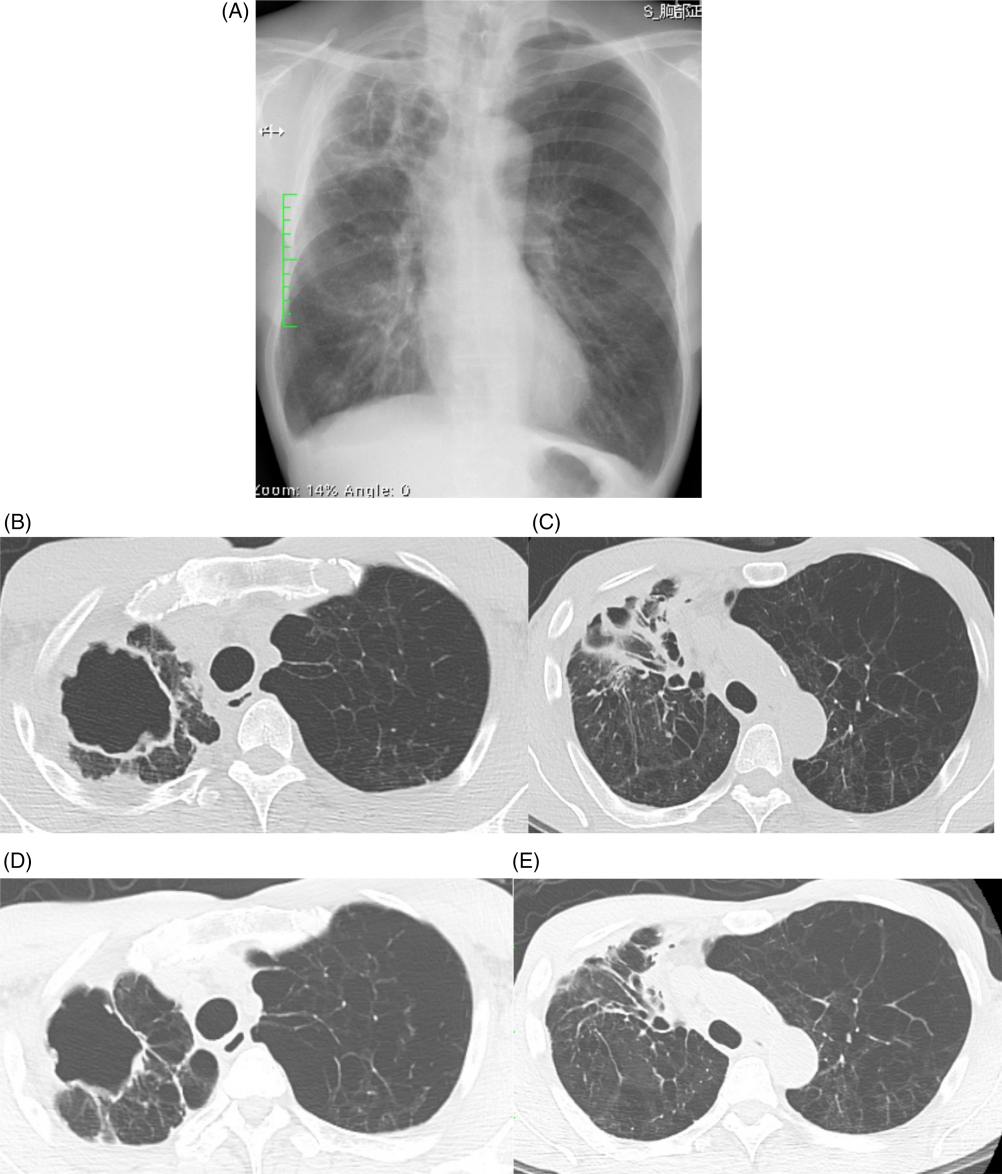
The chest X‐ray on the first visit demonstrated the large cavitary lesion in the right upper lung fields (A). The thoracic computed tomography showed the large, thin‐wall cavity lesion, the thickness of pleural wall, trabecular and linear shadows in the upper right lobe, and bronchiectasis in the upper and middle lobe (B, C). Six months after treatment, the cavitary lesion and trabecular shadows were decreased (D, E).
